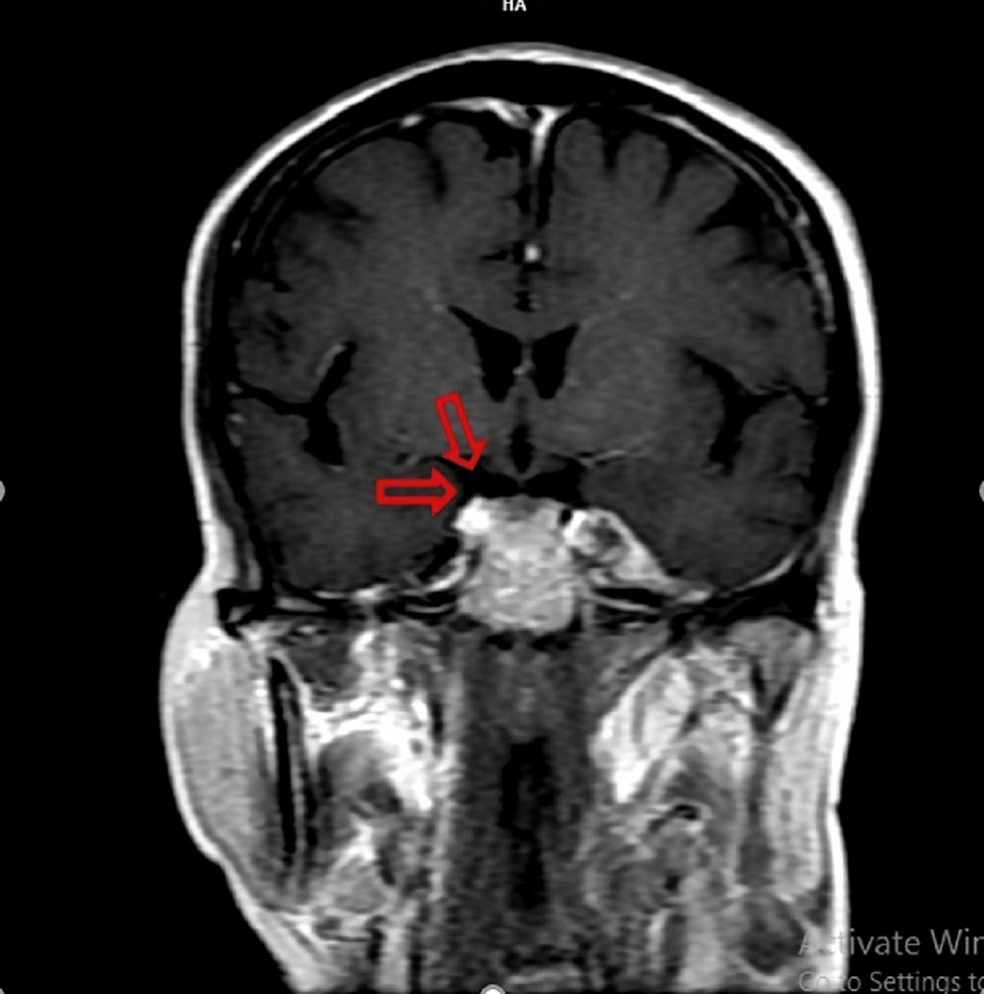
MRI brain (coronal view) showing intense post-contrast enhancement (arrow) in left cavernous sinus s/o metastasis
